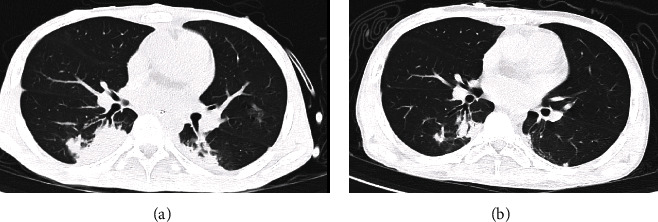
Comparison of computed tomography scan of the chest showed improvement in the 6^th^ week after voriconazole treatment. (a) Two weeks post-treatment: consolidation observed in both lower lobes, with greater prominence in the right lower lobe. (b) Six weeks post-treatment: significant resolution of consolidation in the right lower lobe.
